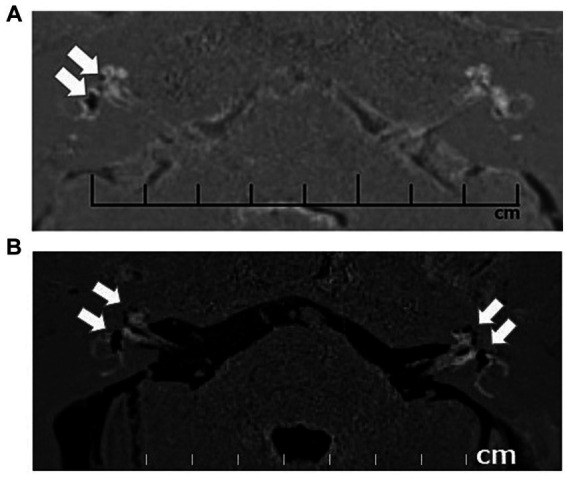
Contrast-enhanced magnetic resonance images (CE-MRI) of unilateral and bilateral endolymphatic hydrops. Unilateral (right) endolymphatic hydrops (A) and bilateral endolymphatic hydrops (B). Arrows indicate the endolymphatic hydrops in the cochlea and vestibule.
